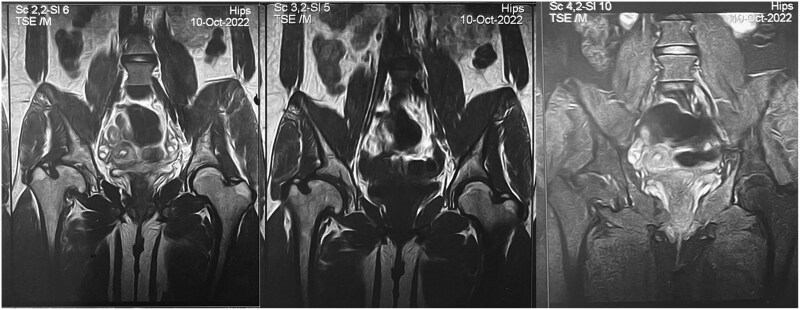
MRI images show right femoral head deformity with signs of avascular necrosis, including coxa magna, coxa vara, and a short neck. The femoral head is partially contained with acetabular remodeling. No labral tears, cysts, hip effusion, or synovial thickening. Both SIJ and lower lumbar vertebrae are normal.
